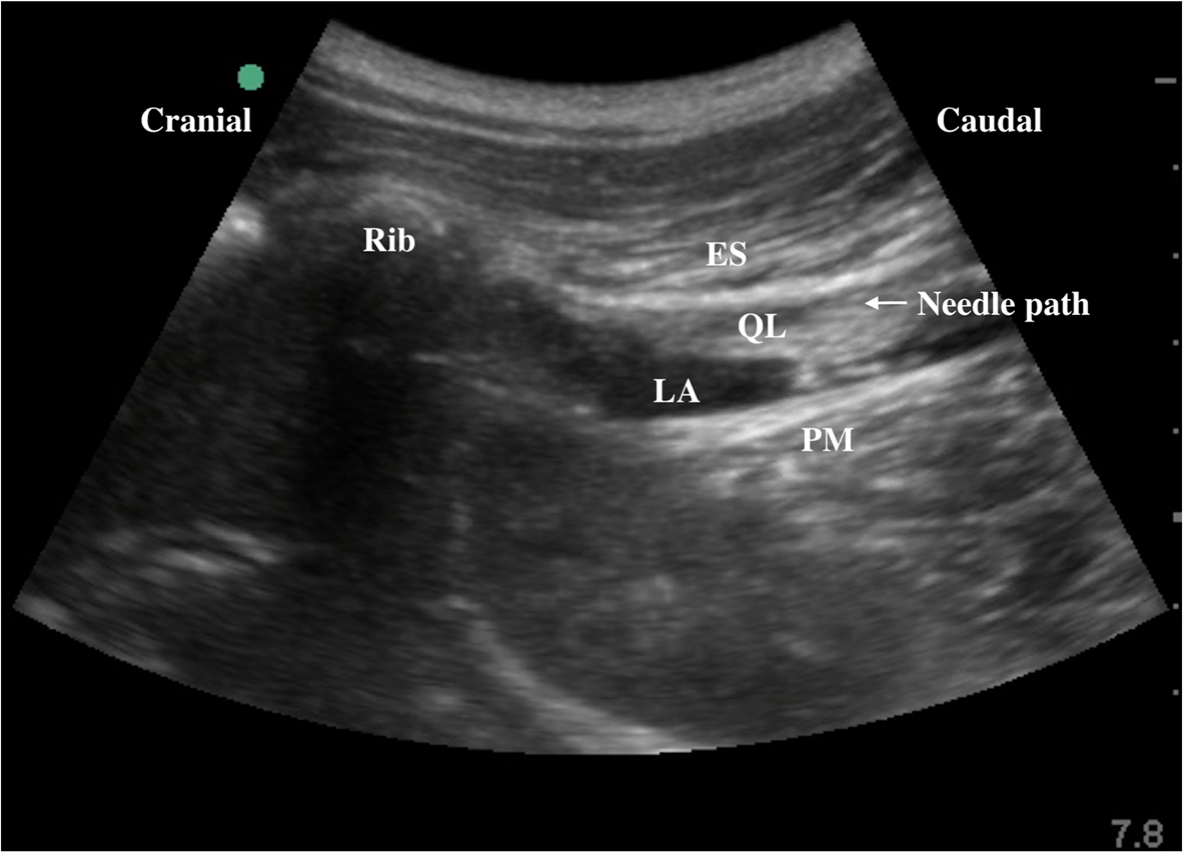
Ultrasound image of the subcostal approach to transmuscular QLB. LA spread betweem QL and PM muscle. *LA* local anesthetic, *QL* quadratus lumborum muscle, *ES* erector spinae muscle, arrow shows the needle path
